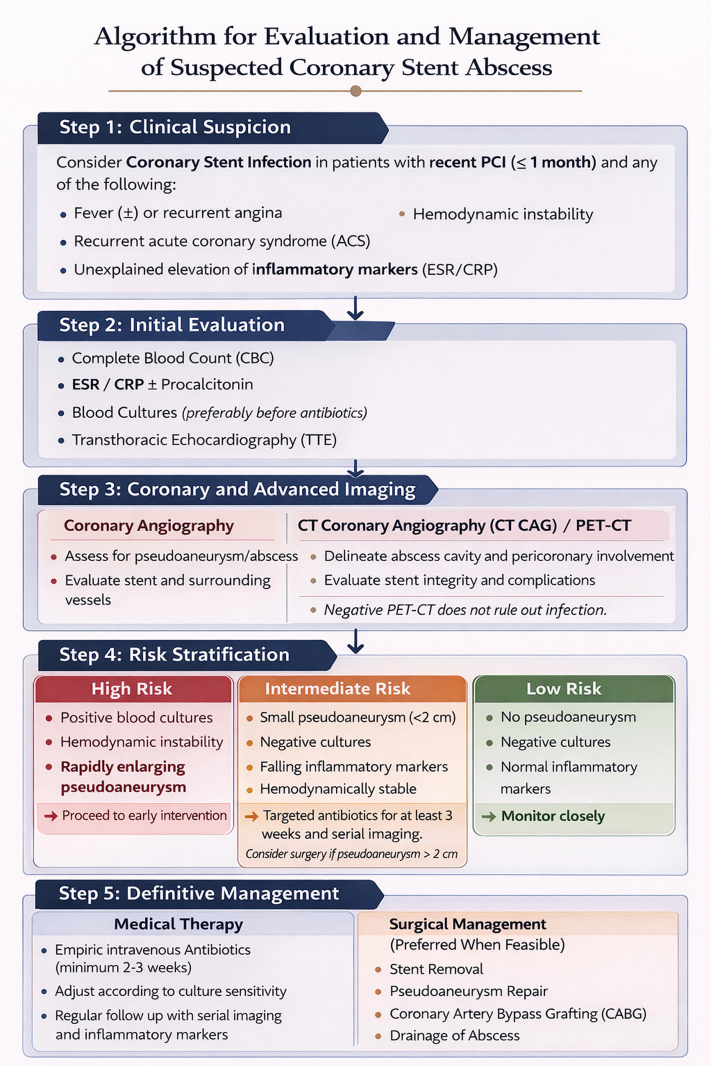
Proposed Diagnostic and Management Pathway in Coronary Stent Abscess Patients with recent PCI presenting with fever, recurrent ischemia, or new pseudoaneurysm should undergo structured evaluation including blood cultures and coronary imaging. Negative PET imaging result does not exclude infection. Prolonged targeted antimicrobial therapy and timely surgical source control represent the preferred management strategy, with percutaneous exclusion reserved for selected high-risk patients. CRP = C-reactive protein; CT = computed tomography; ESR = erythrocyte sedimentation rate; PCI = percutaneous coronary intervention; PET = positron emission tomography.
